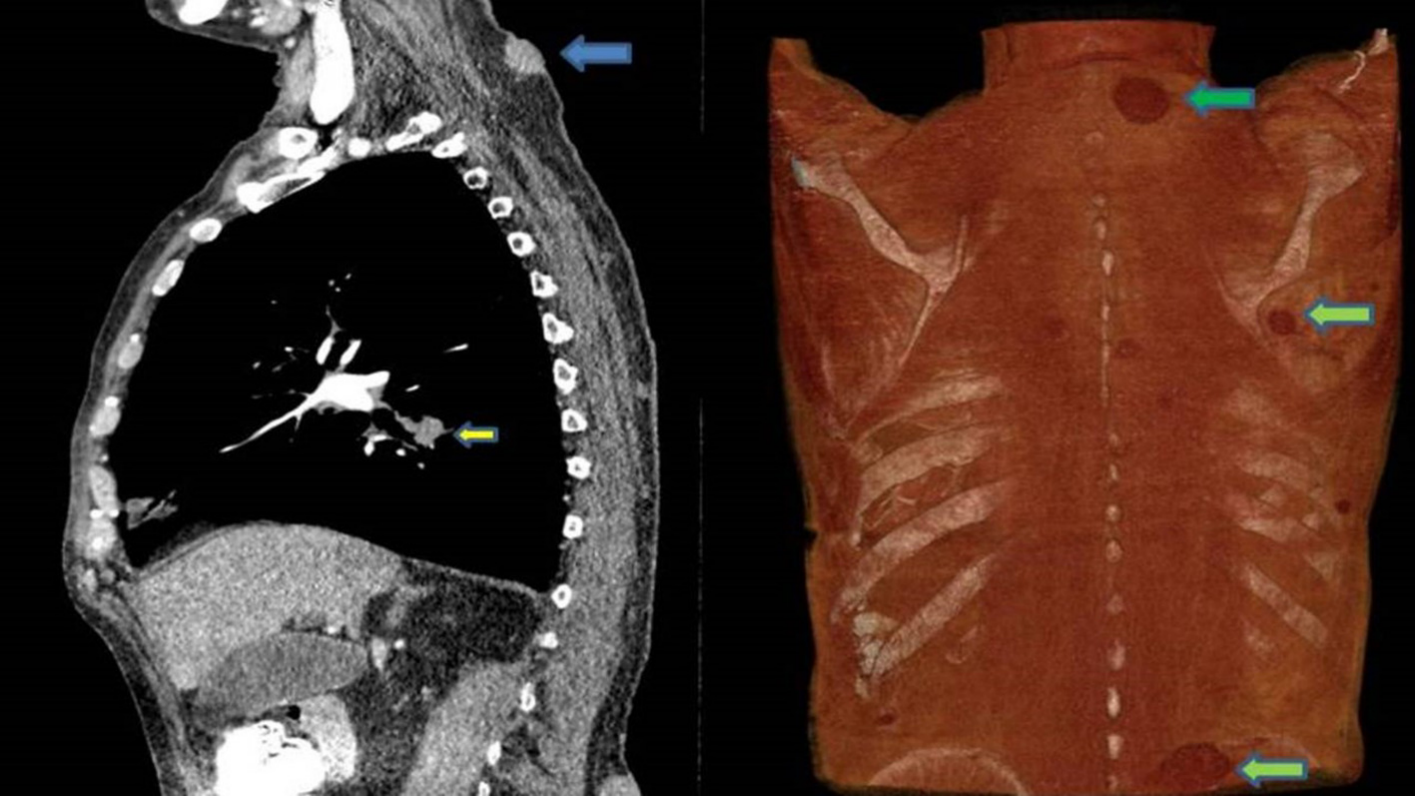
CT-TAP shows 2 x 2 cm mass in right lung lower lobe, 3 x 3cm soft tissue mass at C6-C7 level posteriorly and 4 x 2 cm mass on lumbar region.
